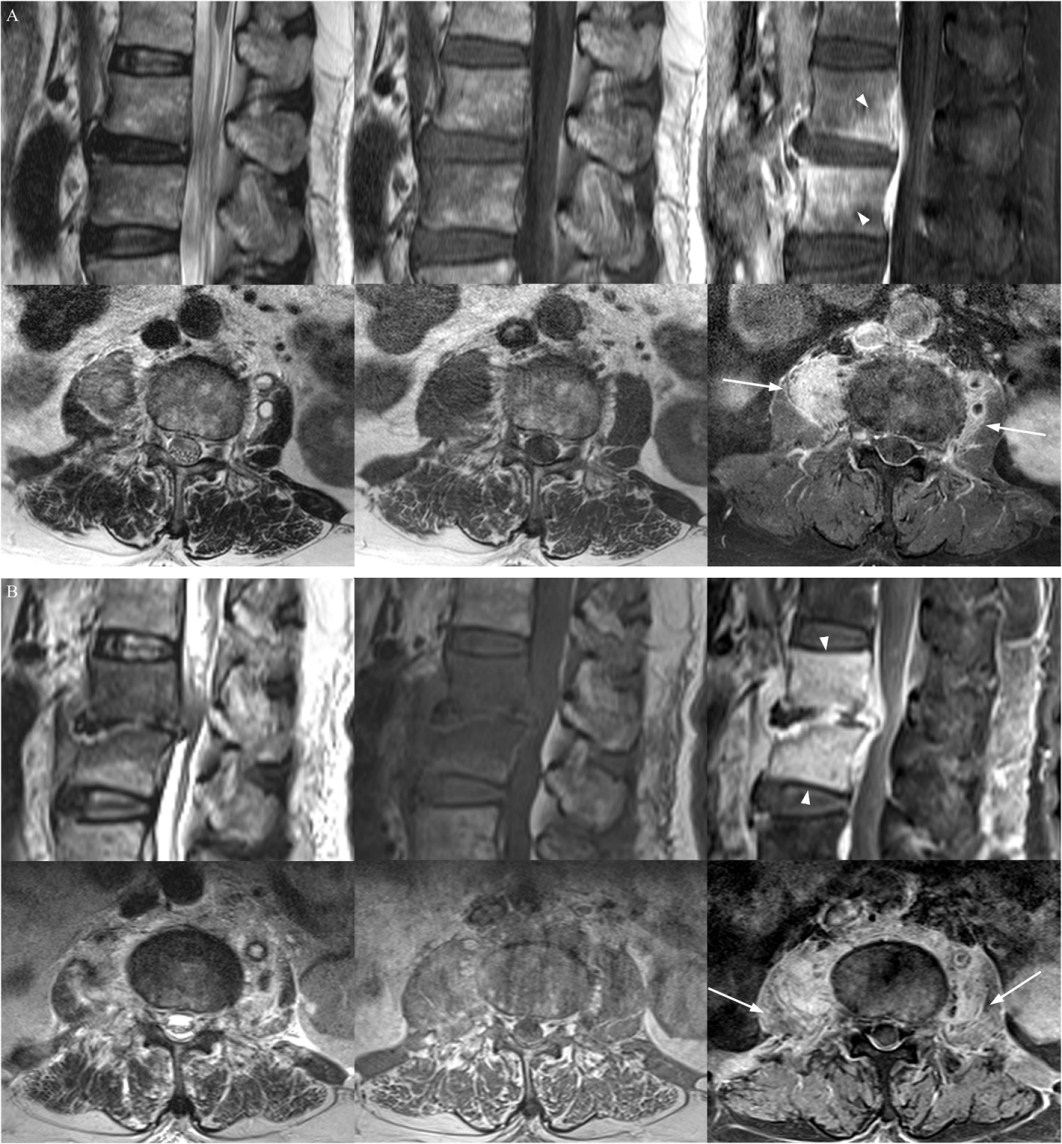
A patient with spondylodiscitis at L2–3 (*Klebsiella pneumoniae*, follow-up interval 40 days). T2-weighted (left), T1-weighted (middle), and contrast-enhanced fat suppressed T1-weighted images (right) of the spine. Comparing contrast-enhanced fat suppressed T1-weighted sagittal (upper) and axial (lower) images of baseline (a) and follow-up (b), bone (arrow heads), soft tissue (arrows), and disc findings all worsened. CRP was resolving (130.9 to 57.5 mg/L [0.0–5.0]) and ESR was worsen (52 to 68 mm/hr. [0–15]) at baseline and follow-up, respectively
